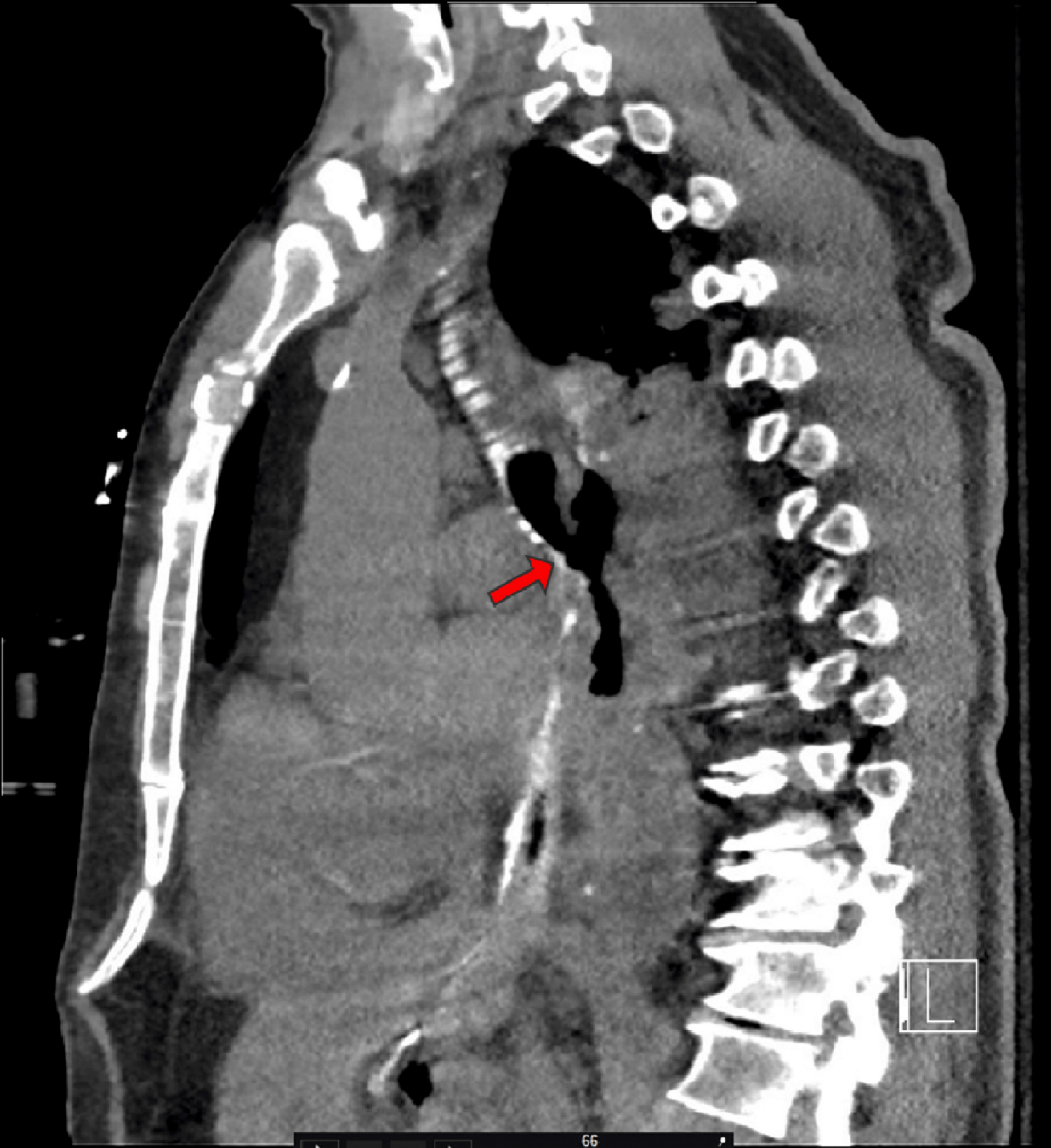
Sagittal view computed tomography (CT) of the thorax demonstrating the bronchoesophageal fistula (BEF) (indicated by the red arrow)
